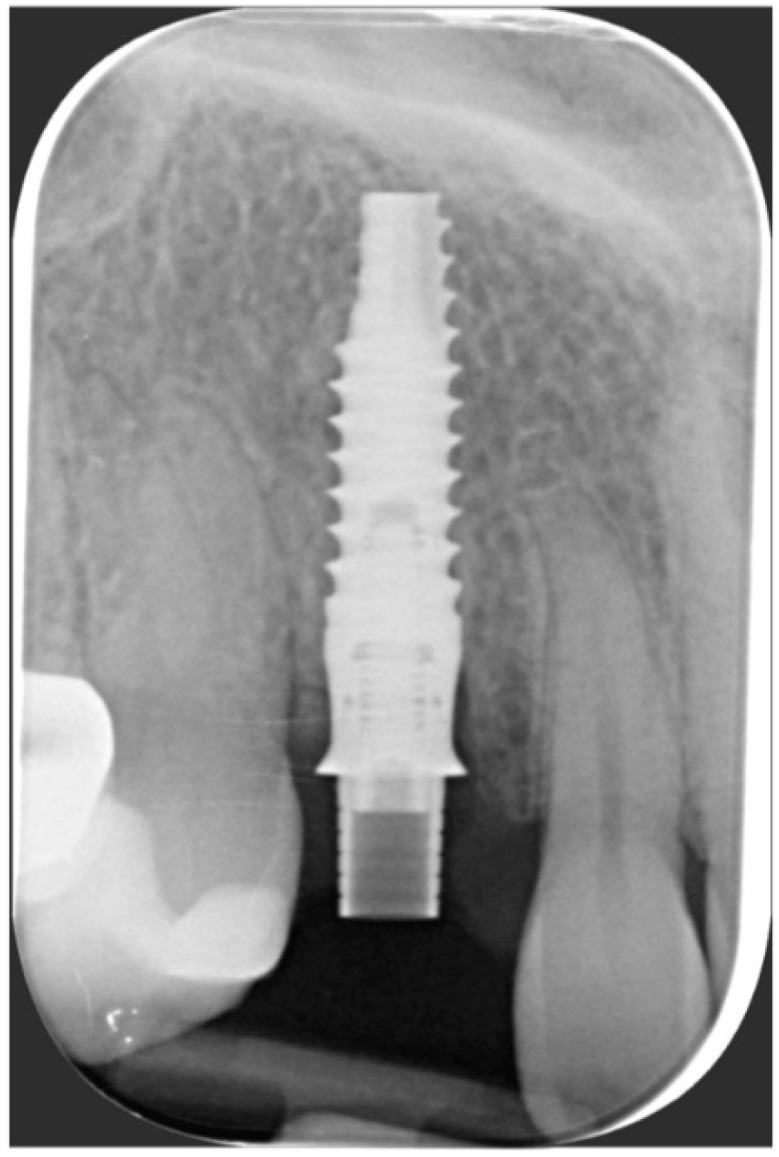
Radiographic evaluation following implant placement.
